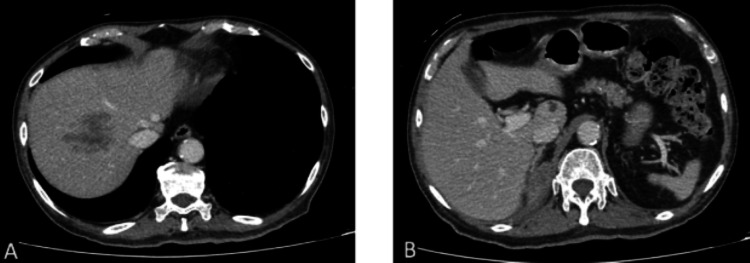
Axial CT images: (A) An ill-defined, heterogeneous hypodense lesion in segment VIII. (B) A smaller, ill-defined, heterogeneous hypodense lesion in segment I.
